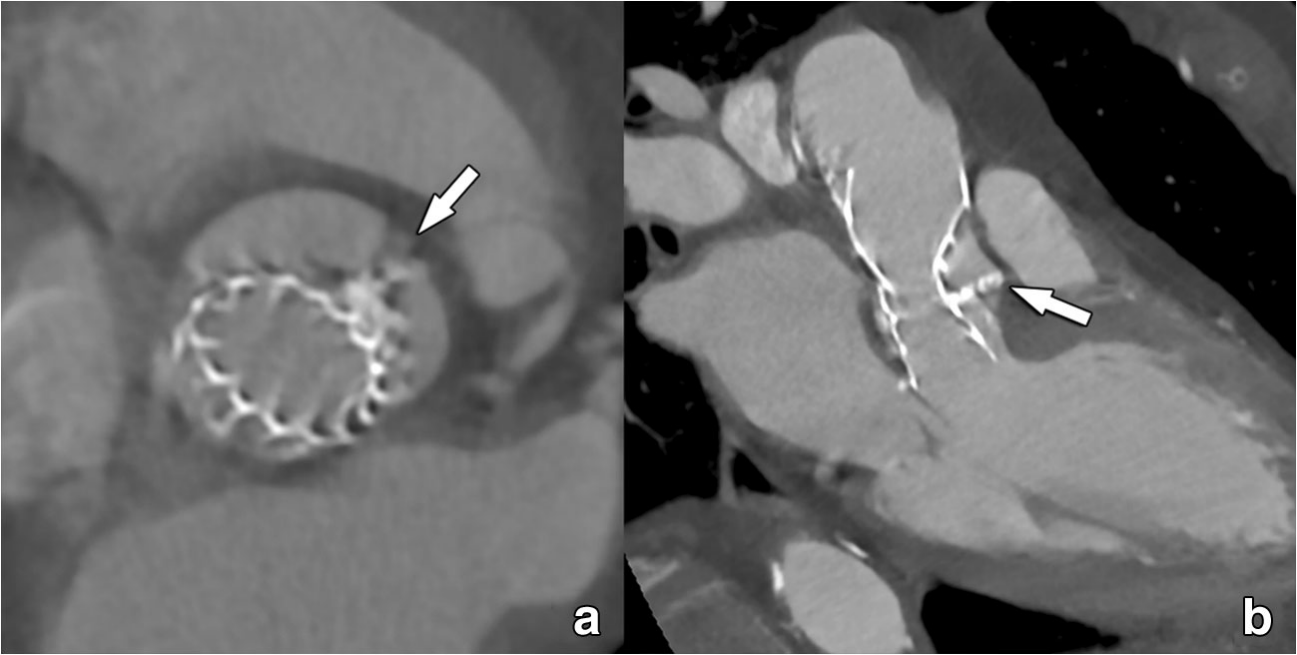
Incomplete and asymmetric deployment of a self-expandable THV due to interposition of extensive native leaflet calcifications (arrow in a, b) between the prosthetic valve and the wall of the aortic sinus. Severe calcifications can complicate prosthesis deployment as in this case, leading to a deformed THV. Nevertheless, caution should be taken when extrapolating morphological findings into a potential dysfunction. While in this case the residual gap between the THV and the aortic wall would suggest a severe paravalvular leakage, this was not the case on Doppler echocardiography examination, with the calcification apparently acting as an additional seal. Valvular function was acceptable, and no further intervention was deemed necessary
